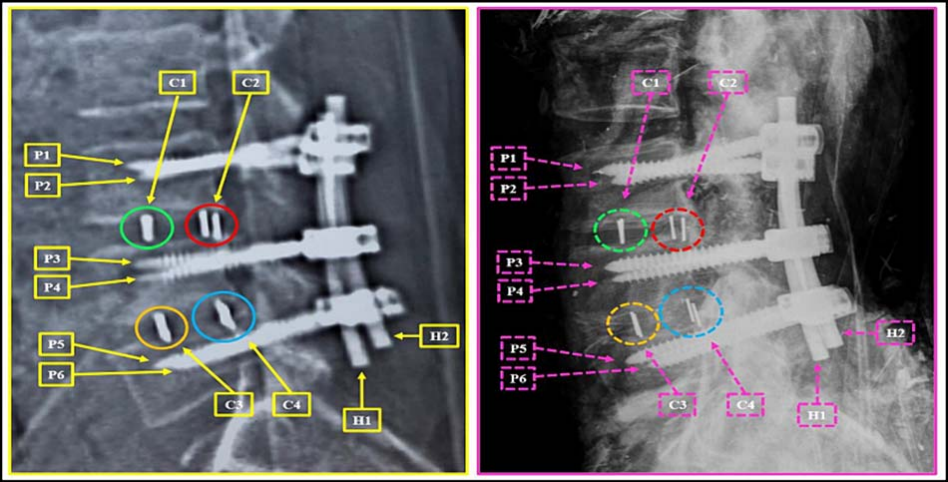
Comparison between the antemortem CT images (left) and postmortem lateral X- ray (right). Elements of agreement.
